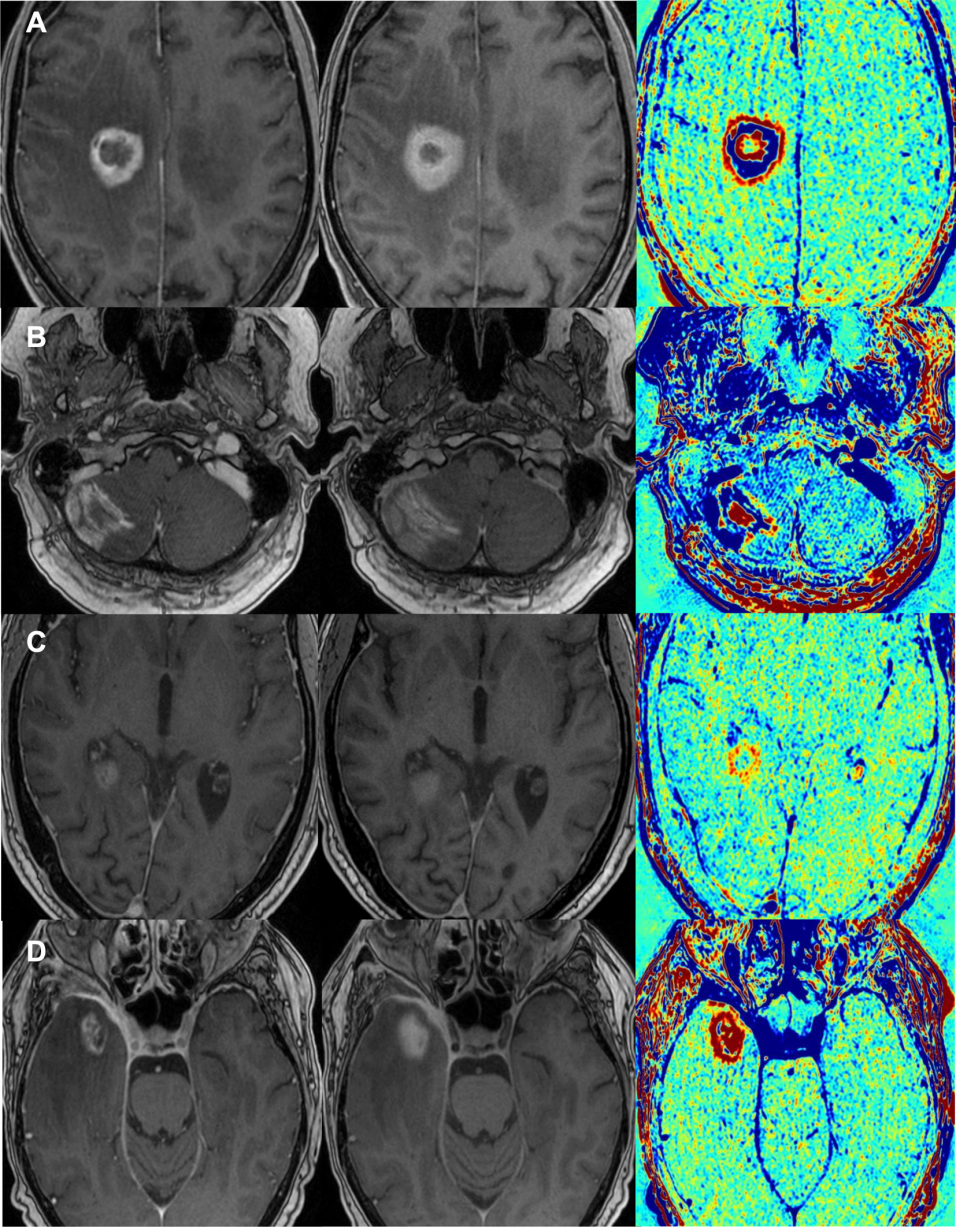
Examples of contrast clearance analysis (CCA). The images show four different patients (A-D), each with a regular contrast-enhanced T1-MRI sequence, a late phase T1-sequence ∼1 h after contrast media application, and their CCA (from left to right). Tumor tissue is depicted as blue in the CCA, while reactive tissue is depicted as red. (A) Glioblastoma (WHO 2016 grade IV) IDH wt: a frontoparietal lesion showing tumor tissue in a circular formation with reactive components centrally and at the lesional border (Patient ID 17). (B) Lung adenocarcinoma with brain metastases: a right cerebellar lesion showing tumor tissue with reactive components in the surrounding area (Patient ID 03). (C) Glioblastoma (WHO 2016 grade IV) IDH wt: a periventricular lesion showing spotted areas with reactive tissue (Patient ID 20). (D) Maxillary squamous cell cancer with brain infiltration: a lesion in the right temporal lobe consisting nearly entirely of reactive tissue (Patient ID 25). IDH, isocitrate dehydrogenase; MRI, magnetic resonance imaging; WHO, World Health Organization; wt, wild type.
